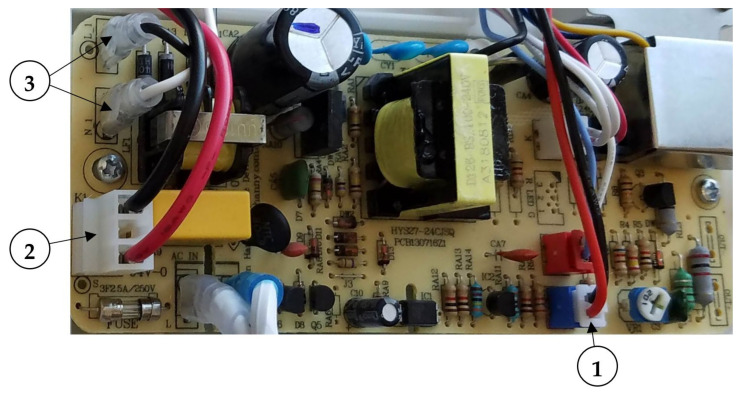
Plug locations for modification to main circuit board for the ultrasonic humidifier: 1. Speed control; 2. Power switch; 3. 120VAC connected to 5VDC power supply.
